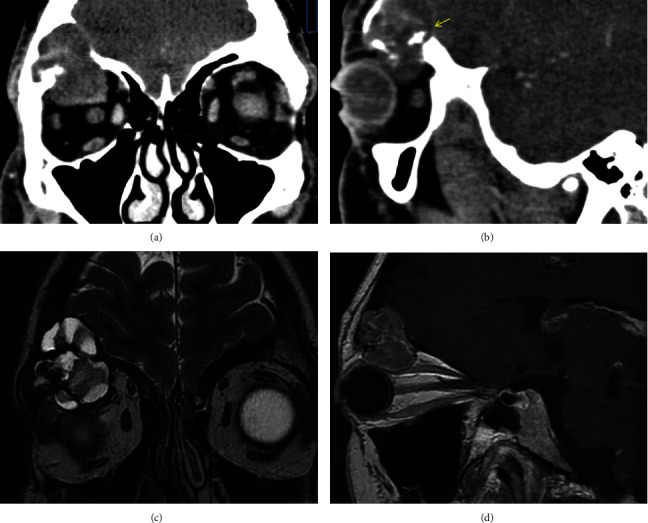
(a, b) Contrast-enhanced coronal and sagittal CT images, demonstrating an expansive lesion arising from the roof of the right orbit with a heterogeneous enhancement and soft tissue attenuation. Inside view of the lesion reveals several small foci of mineralization (arrow). The lesion shows the osseous expansive changes, with thinning of superior and inferior wall of the roof which leads to an invasion over the frontal sinus and into the orbit. (c) Coronal T2-weighted MRI shows a well-defined lesion with a low-signal-intensity margin representing either osseous sclerosis or a pseudocapsule. The lesion shows a multilobulated lytic pattern which reveals markedly increased signal intensity, reflecting the expansive cystic component, and low signal intensity in the small solid regions. (d) Sagittal postcontrast T1-weighted MR image shows a well-encapsulated mass with homogenous contrast enhancement.
